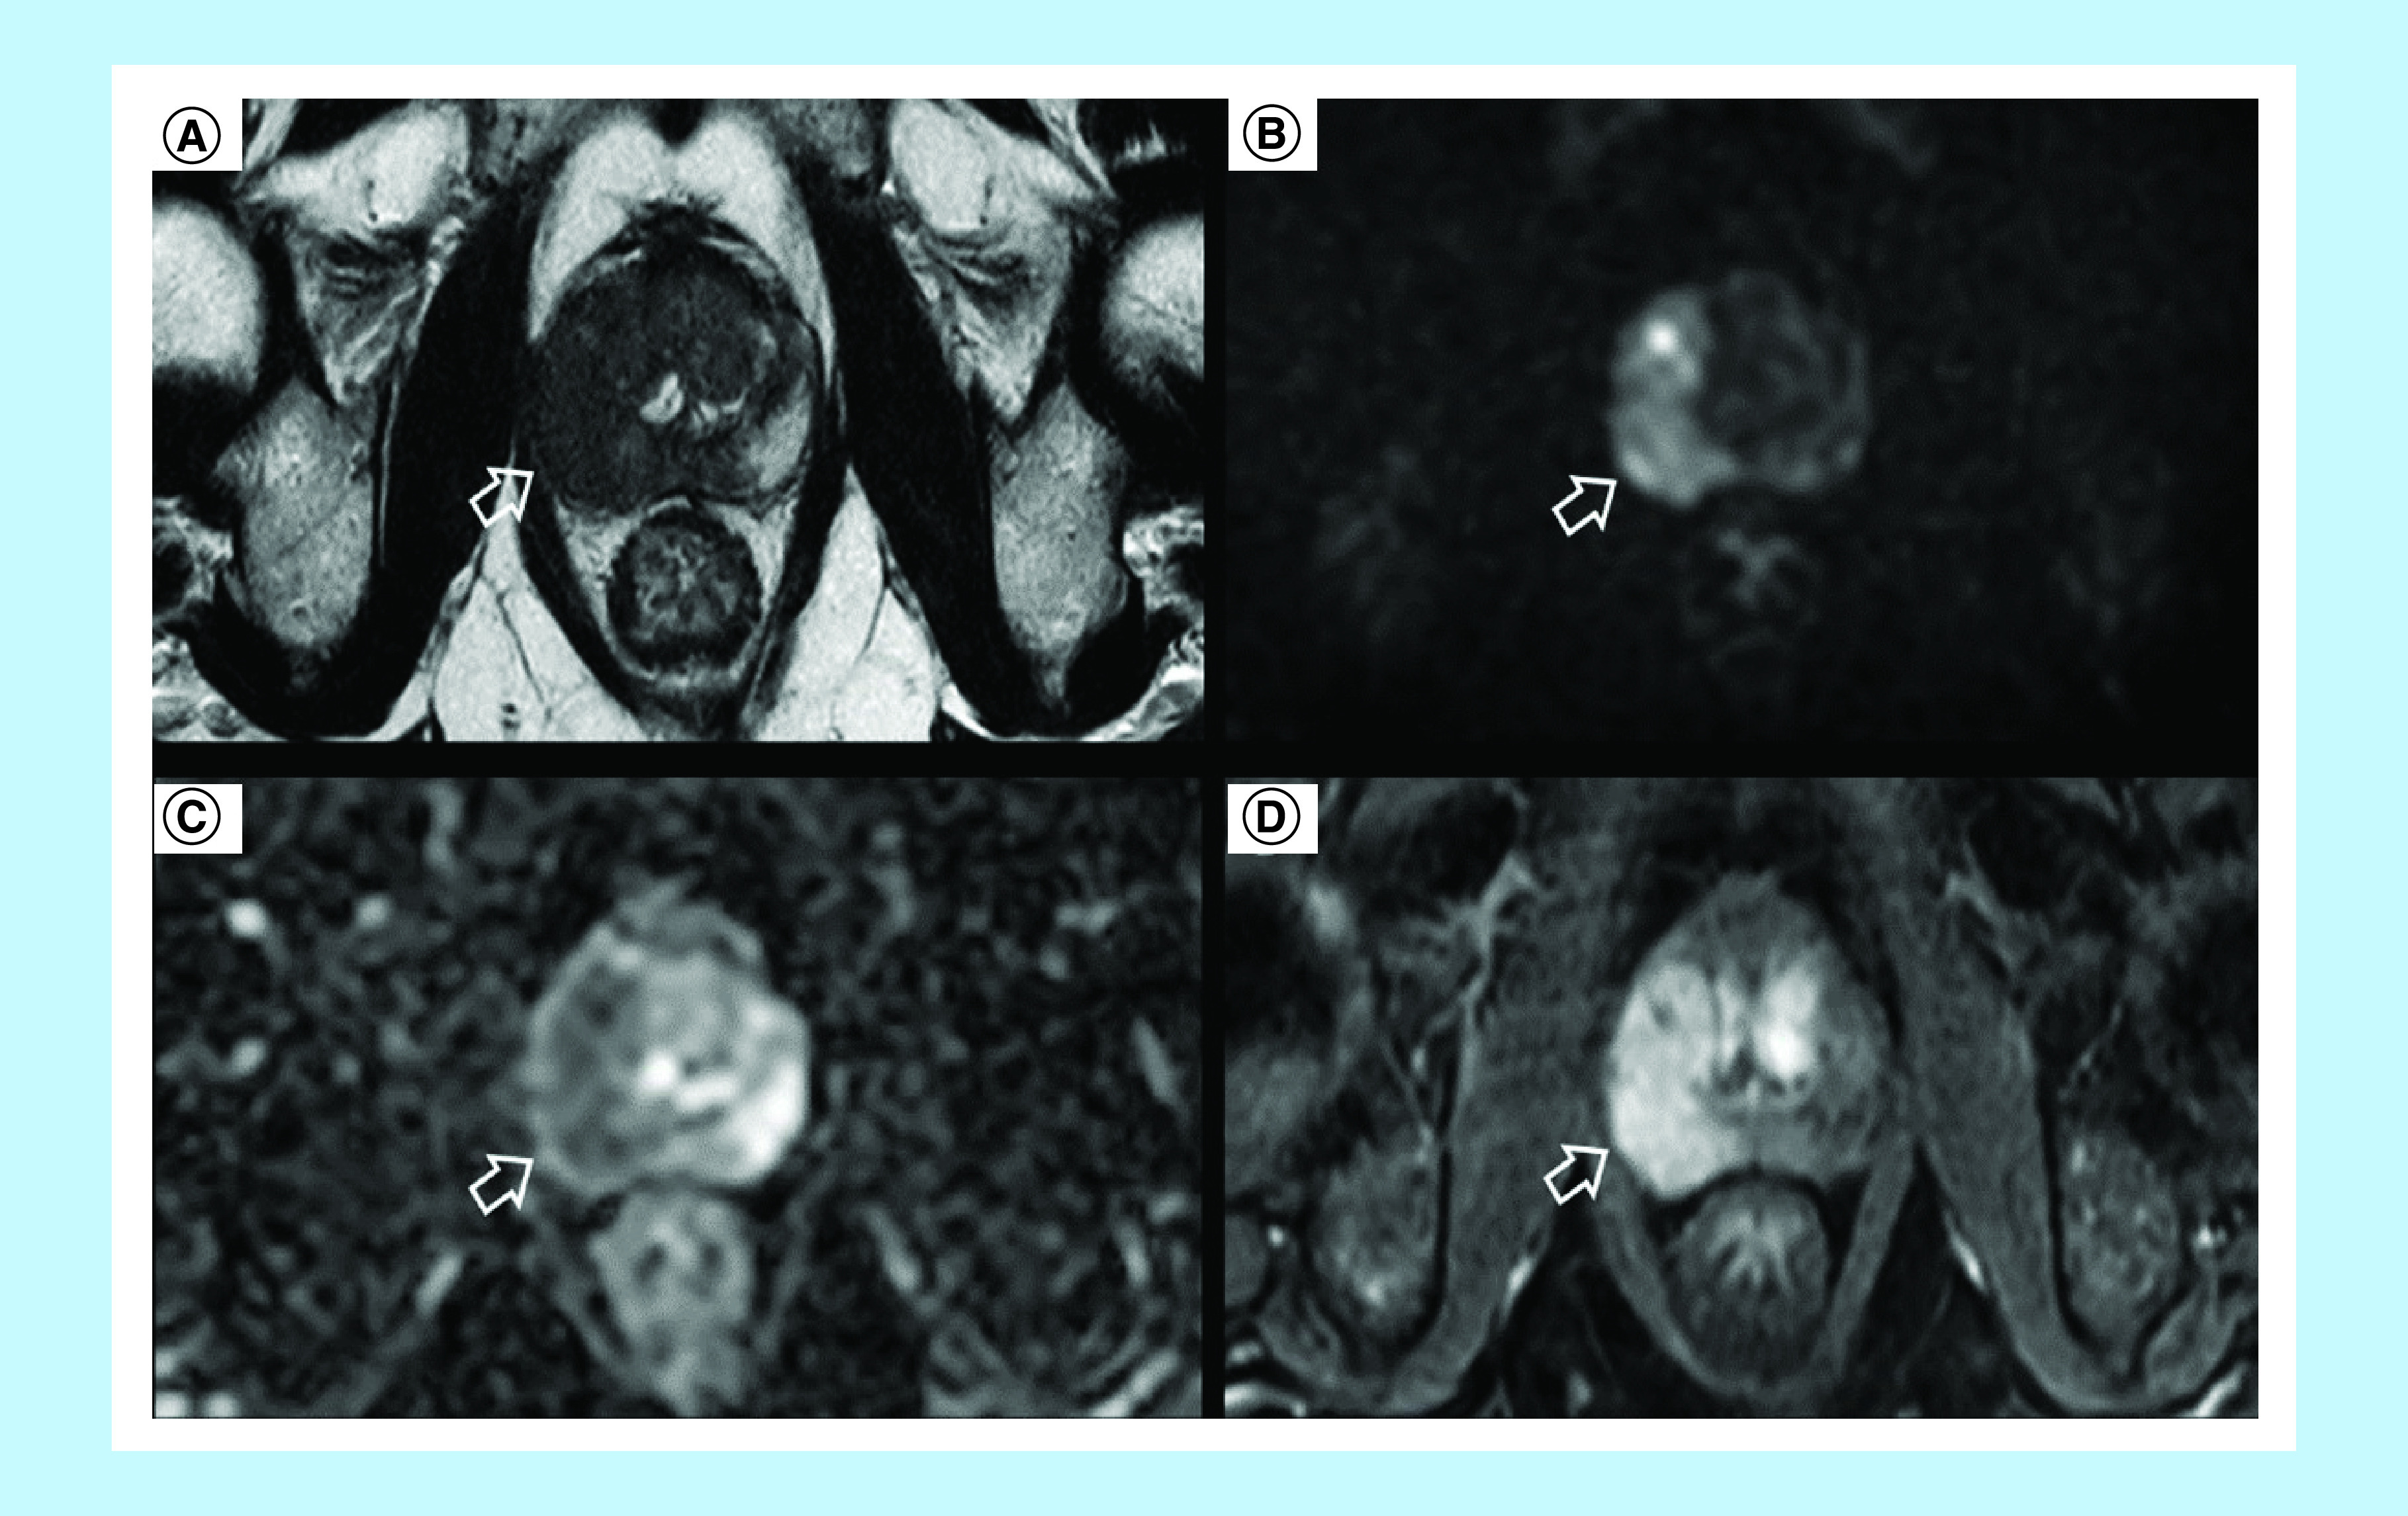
Multiparametric magnetic resonance imaging findings. (A) Axial T2WI scan demonstrating a large (4 cm) nodular
